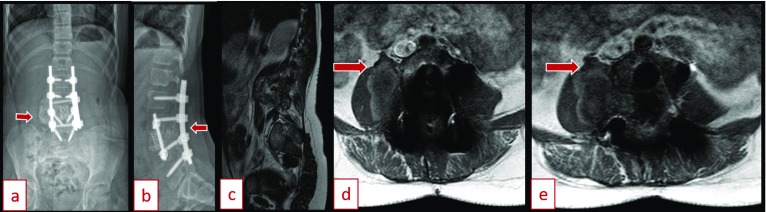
(a) EOS (Low dose radiation imaging device) spine PA view preoperative (February 2017). (b) Spine lateral view preoperative (February 2017). (c) Preoperative sagittal MRI demonstrating recurrent osteosarcoma (December 2016). (d, e) Preoperative axial MRI demonstrating recurrent osteosarcoma proximity to neurovascular structures (December 2016).
